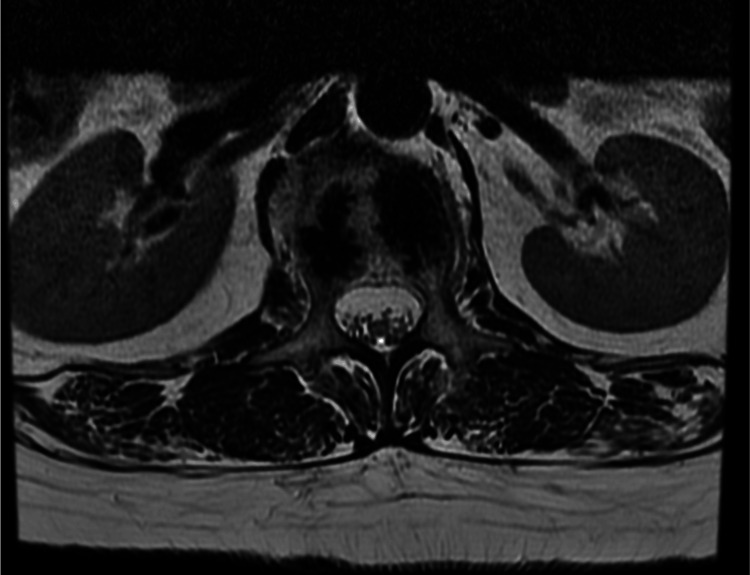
MRI T2 weighted image (axial view) of the lumbar spine obtained at three months following the fracture. No gross signs of a malignant tumor at the L1 vertebra, and/or breaching of the vertebral cortex are apparent.
